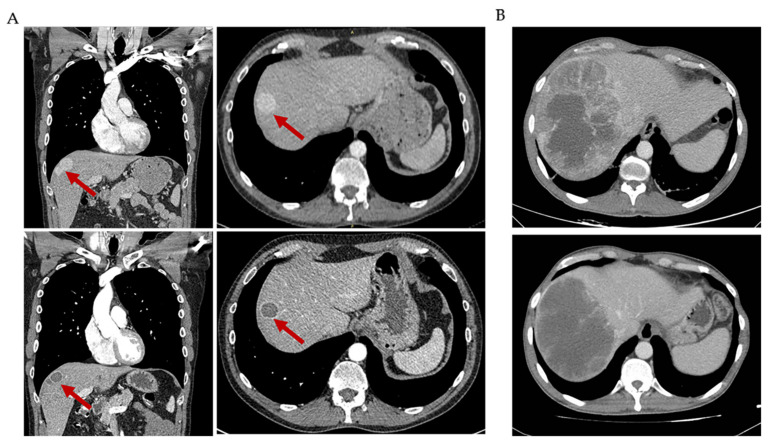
Choi responses to antiangiogenic agents in solitary fibrous tumor (SFT). (A) CT scan of an SFT patient showing a characteristic Choi partial response to pazopanib treatment in one hepatic lesion: decrease in density of 56%. (B) CT scan of an SFT patient showing a characteristic Choi partial response to pazopanib treatment in one hepatic lesion: decrease in density of 33%.
